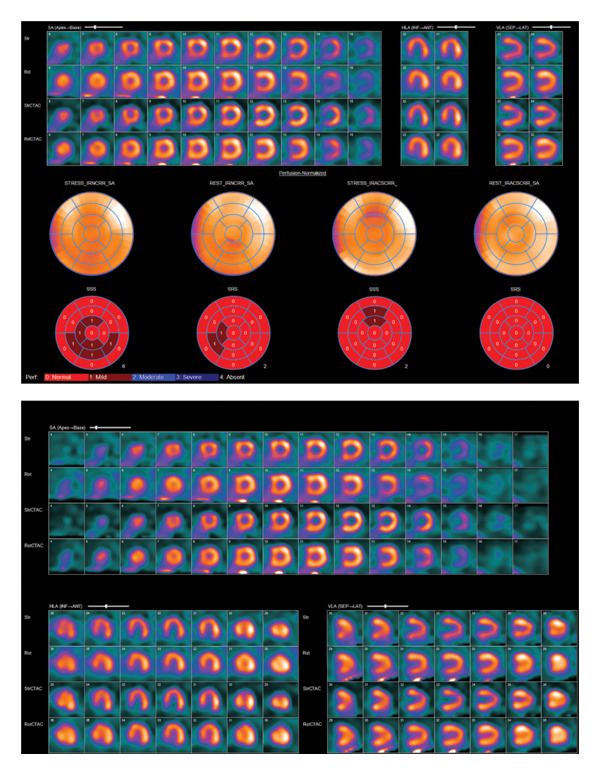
Nuclear SPECT myocardial perfusion test.
